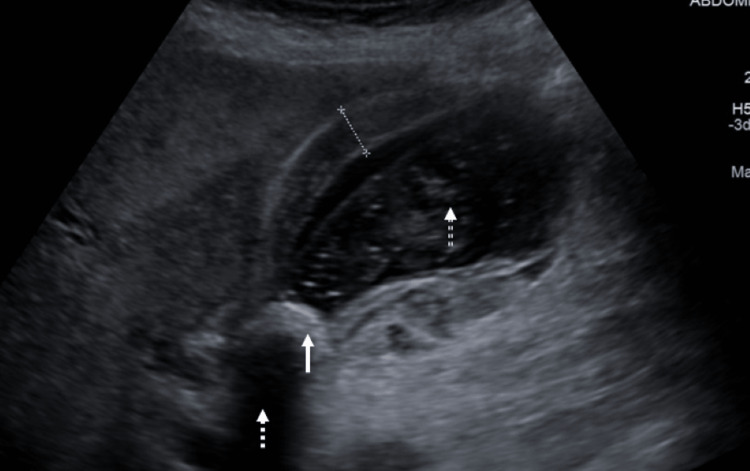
Ultrasound scan at the time symptoms demonstrates gallstone (white arrow) in duodenal lumen with posterior acoustic shadowing (white dashed arrow) and proximal dilation (white double line dashed arrow).
